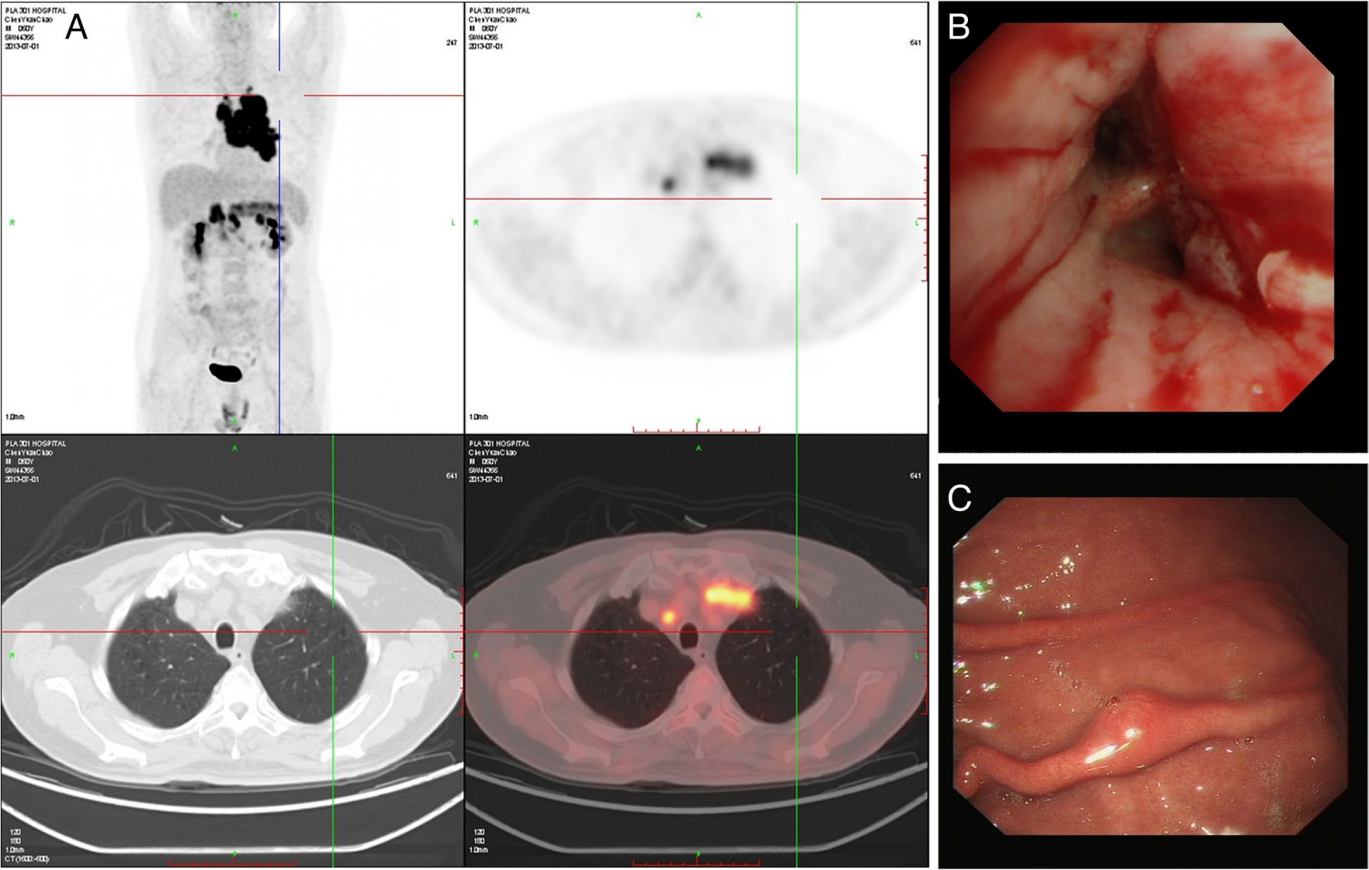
Imaging examination and gastroscopy. A PET-CT examination showed hypermetabolic nodules in the stomach and hilus of the lung. B Bronchoscopy showed a new organism in the opening of the basal segment of the left inferior lobe. C Under gastroscopy, GMNDI showed eminence, nodular or vegetable pattern mass, and ulcer
